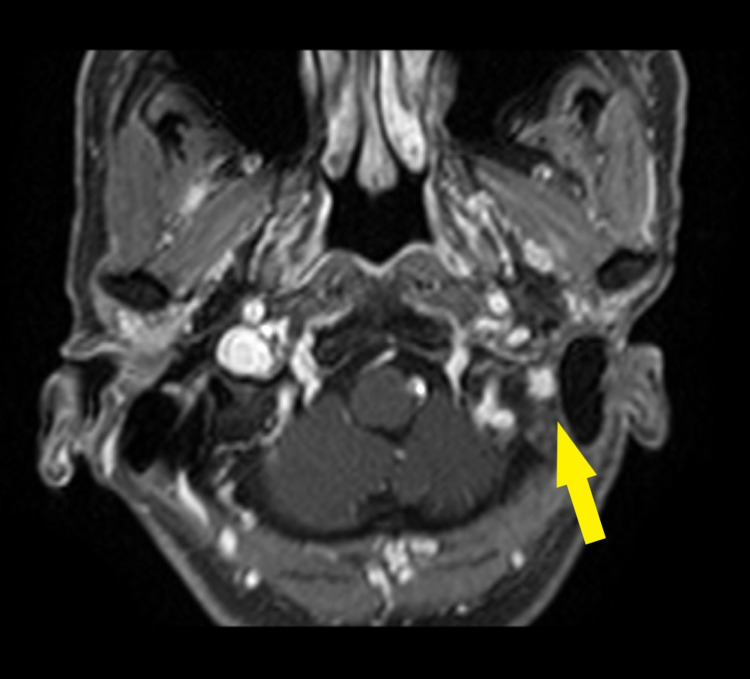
First recurrence in April 2018 Gadolinium-enhanced fat-saturated T1-weighted image Yellow arrow: local recurrence within the radiation field
